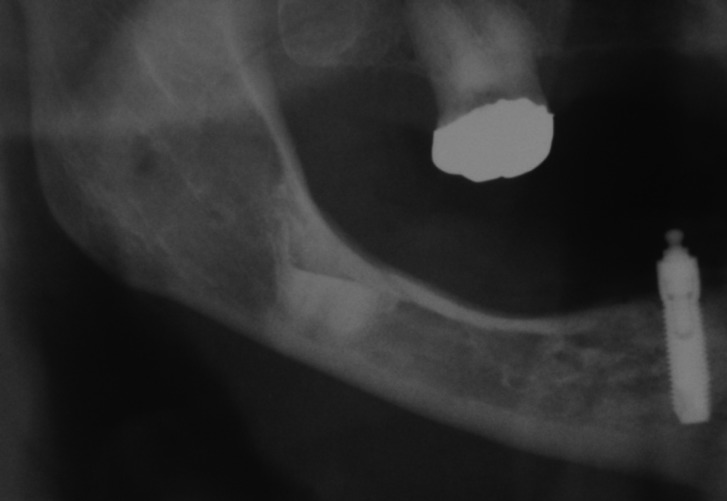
Case 8.Panoramic radiograph taken 3 years later.
